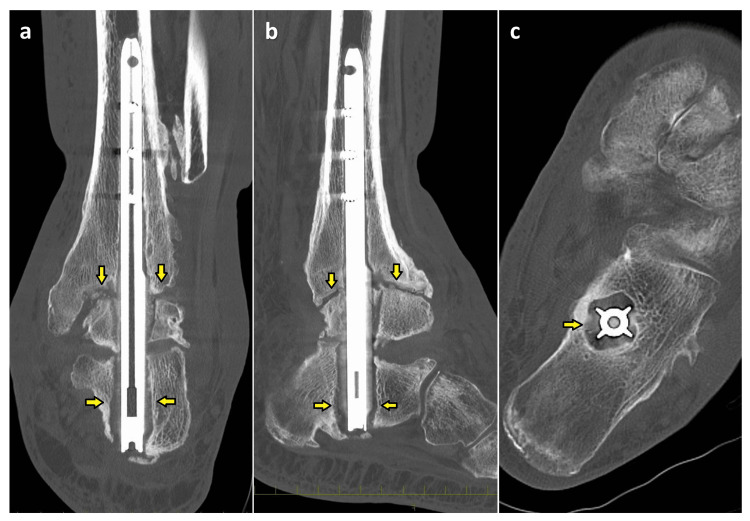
Preoperative CT (a) coronal, (b) sagittal, and (c) axial images CT, computed tomography
